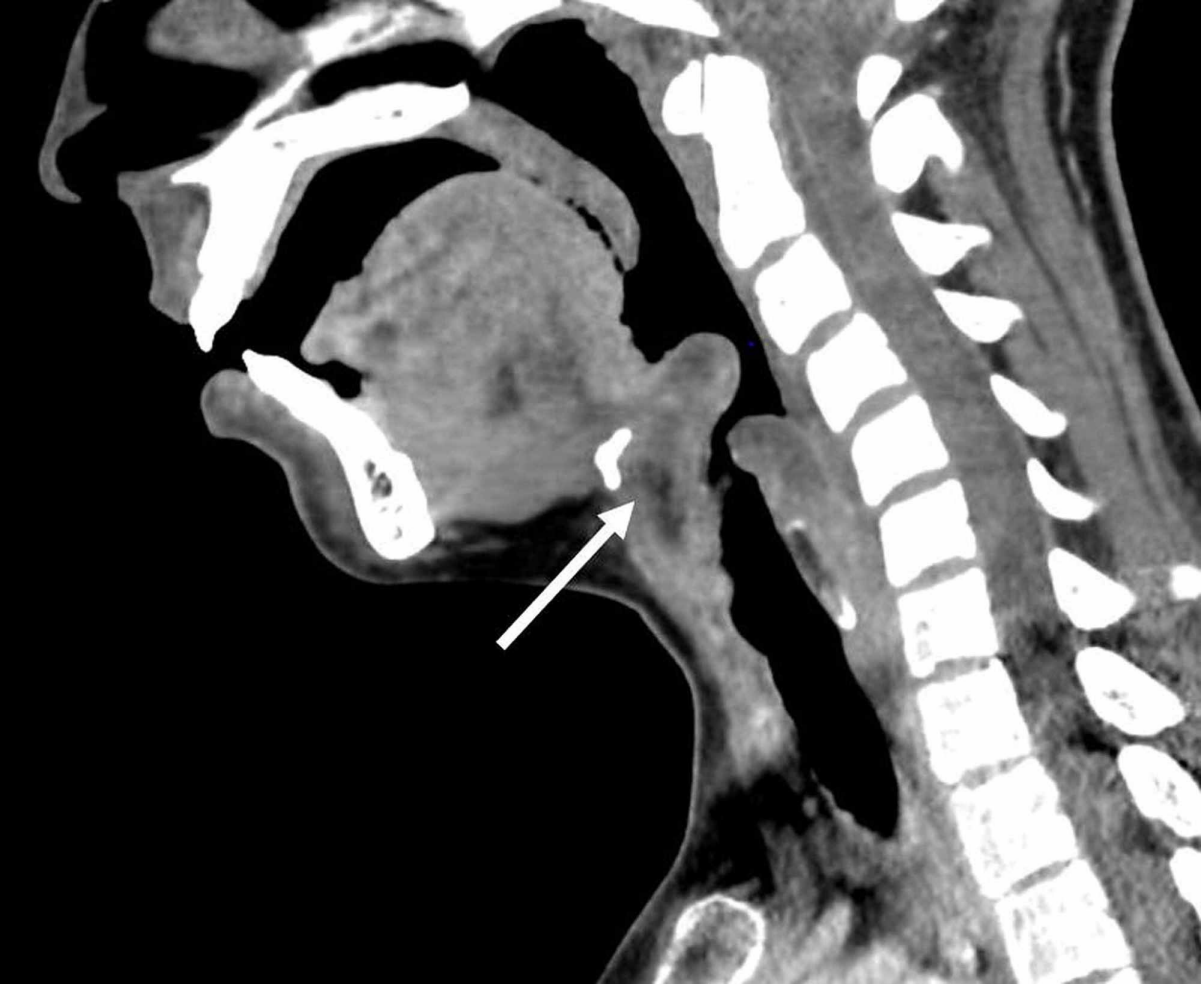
Sagittal computed tomography scan Sagittal computed tomography scan acquired in the emergency room, demonstrating edema of the epiglottis, 1.8 cm abscess formation, and airway compromise.
